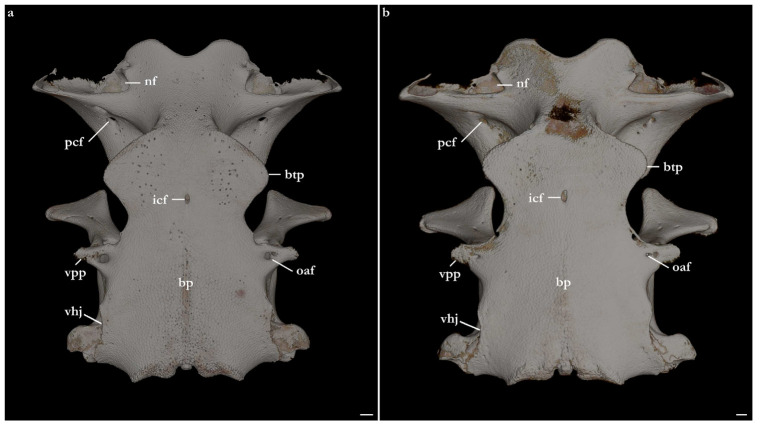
Micro-computed tomography scans of the neurocranium of (a) *Squatina leae* sp. nov., paratype, ZMH 26098, juvenile male, 249.6 mm TL and (b) *S. africana*, ZMH 123064, juvenile female, 309 mm TL in ventral view. Abbreviations: bp, basal plate; btp, basitrabecular process; icf, internal carotid foramen; nf, nasal foramen; oaf, orbital artery foramen; pcf, preorbital canal foramen; vhj, ventral hyoid junction; vpp, ventral postorbital process. Anterior to top; scale bars: 2 mm.
